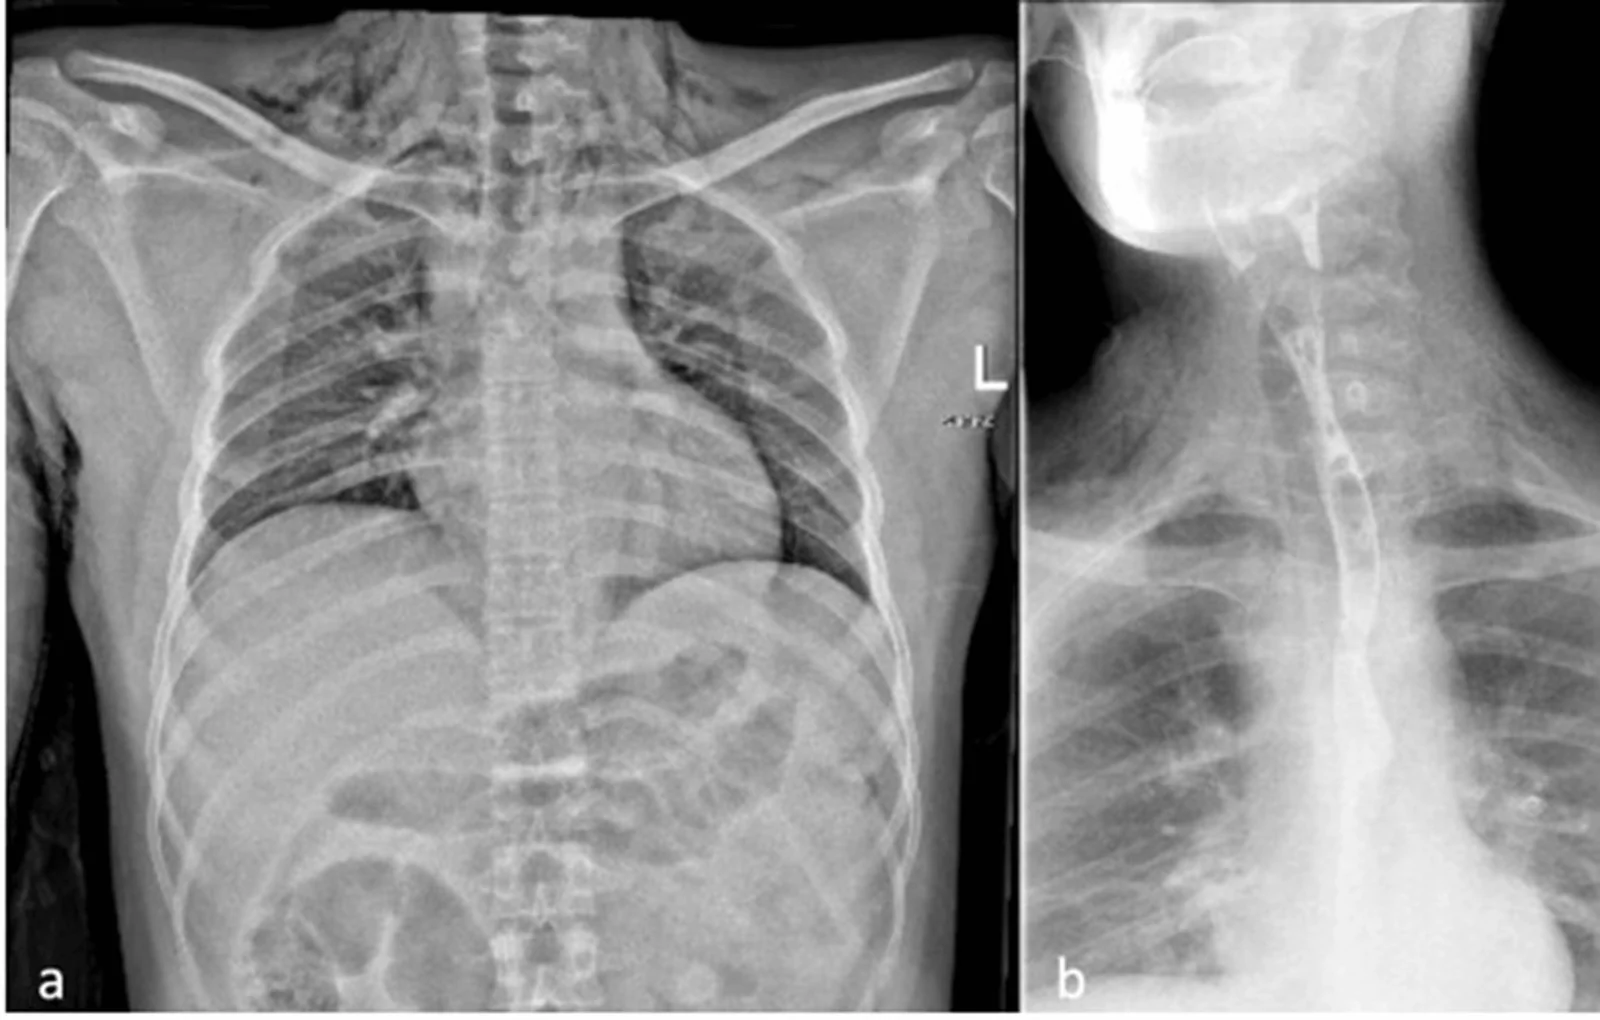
(a) Chest X-Ray: Diffuse emphysematous changes in the superficial and deep muscle planes in the neck. (b) Barium swallow: smooth outline with no filling defects and no contrast leak from the esophagus.
